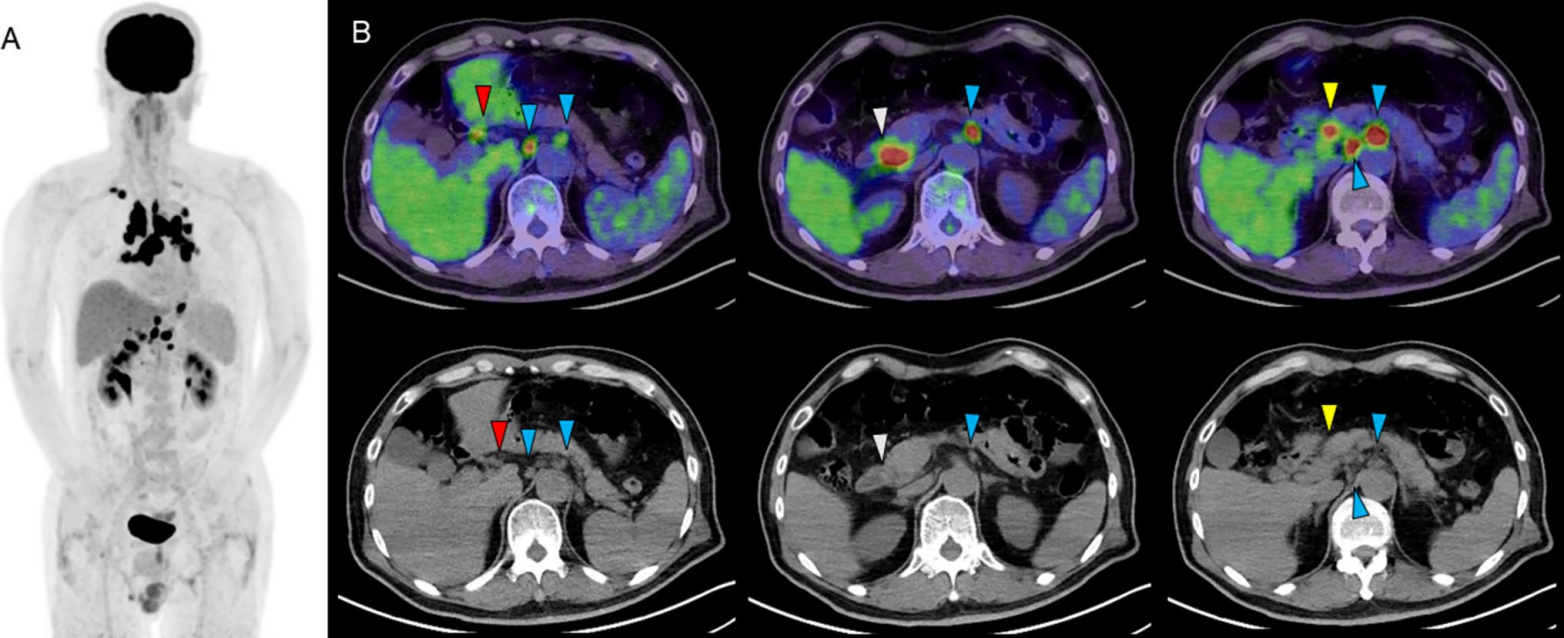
A Maximum intensity projection (MIP) image of FDG-PET, B upper row: fused PET/CT image, lower row: CT portion of PET/CT. The MIP image demonstrates intense FDG uptake in the hilar and mediastinal lymph nodes, as well as in multiple lymph nodes (LNs) in the upper abdominal region. In contrast, no abnormal FDG uptake was observed in the myocardium. Focal FDG uptake was seen in periportal LN (red arrow heads), anterior pancreaticoduodenal lymph node (yellow arrowheads), posterior pancreaticoduodenal LN (white arrowheads), and celiac LNs (blue arrowheads) was seen. Although these lymph nodes are small and exhibit benign morphological features on CT, intense FDG uptake is commonly observed in patients with sarcoidosis
